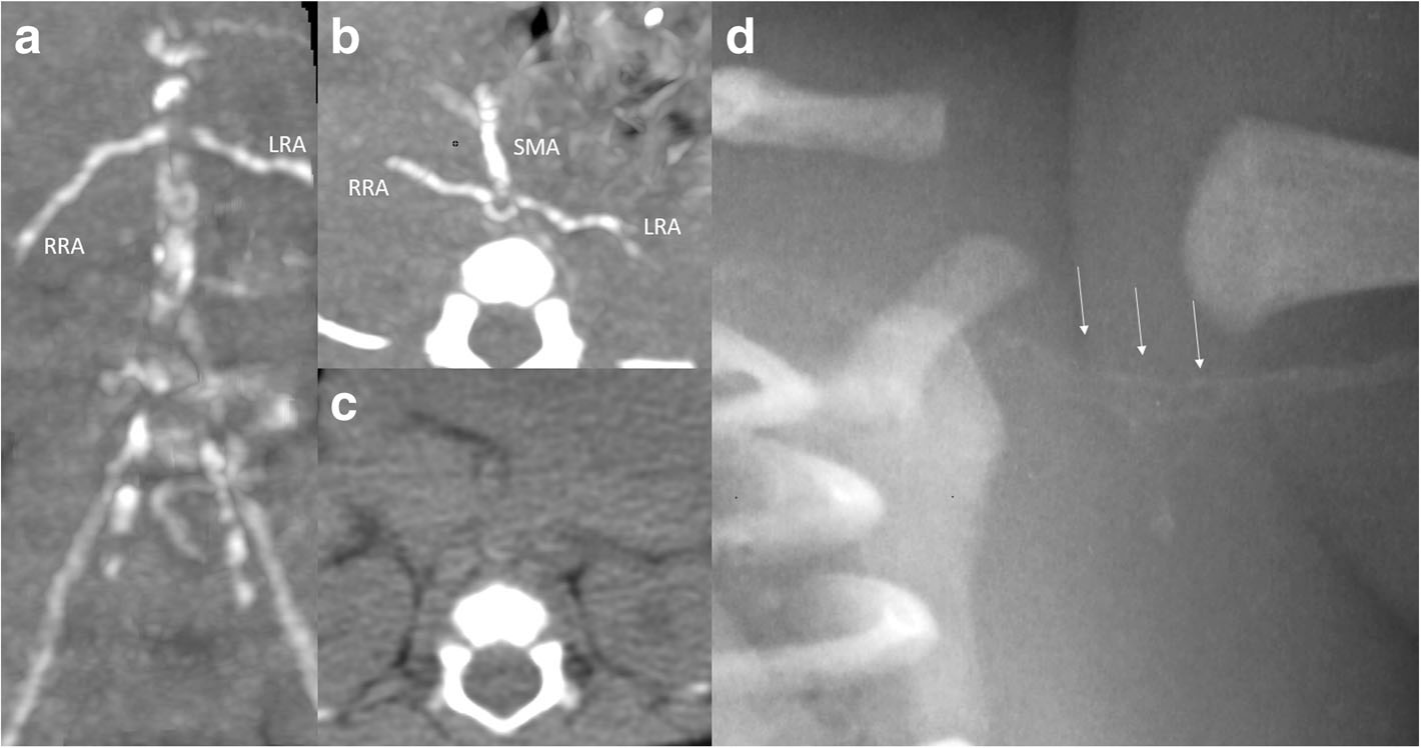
a Abdominal CT-scan frontal view at 1 month of age: huge vascular calcifications of the aorta, the iliac arteries, the renal arteries (RRA: right renal artery, LRA: left renal artery). b Abdominal CT-scan axial view at 1 month of age: calcifications of the superior mesenteric artery (SMA), renal arteries (RRA, LRA) and the aorta. c Abdominal CT-scan axial view at 5 months of age: the arterial calcifications are no longer visible. d Close-up view on the left scapular area of chest X-ray at day 1: subtle calcifications of the left subclavian and left axillary artery (white arrows)
